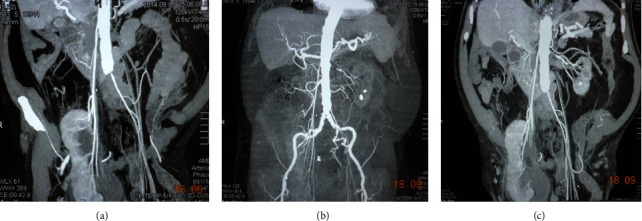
Multidetector-computed tomography. Frontal views present a severely elongated right renal artery and stenosis of the left renal artery (a–c).
